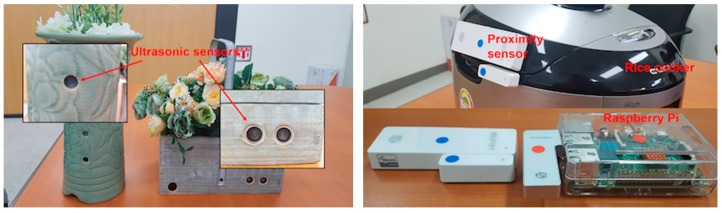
Ultrasonic sensors (left) and rice cooker embedded with proximity sensors (right).
